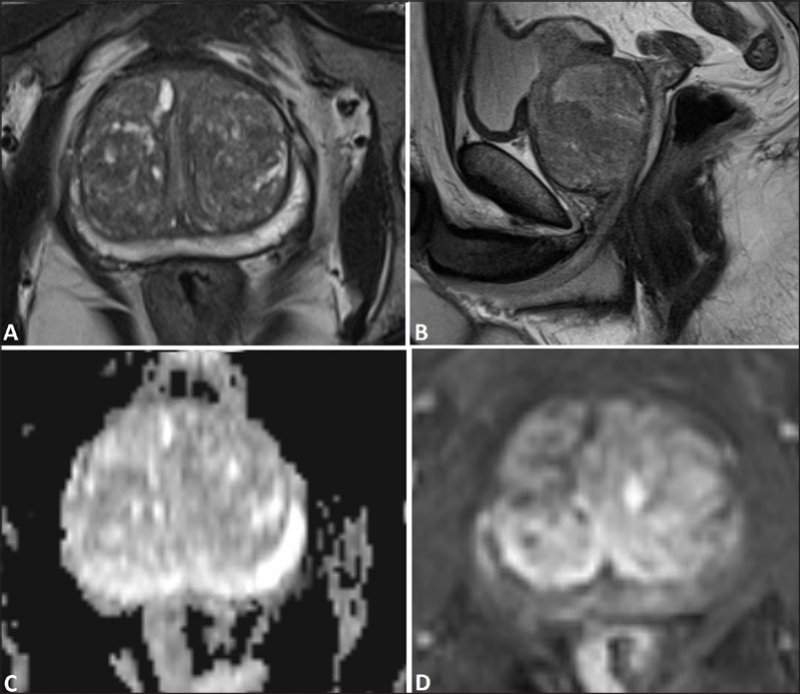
52-year-old man with elevated PSA (12.8 ng/ml). On T2-weighted images (A: axial; B: sagittal) the prostate is enlarged with estimated volume of 151 ml. The peripheral zone shows a normal high signal intensity. On the ADC map of the DWI (C) There are no lesions with restricted diffusion and on the subtraction image of the dynamic contrast enhanced series (D) No suspicious contrast enhancing lesions are demonstrated. The PI-RADS overall assessment score is 1 and no biopsy was performed. The patient was followed clinically and with repetitive PSA samplings for more than 4 years and no clinically significant prostate cancer appeared. The elevated PSA level was caused by benign prostatic hyperplasia.
